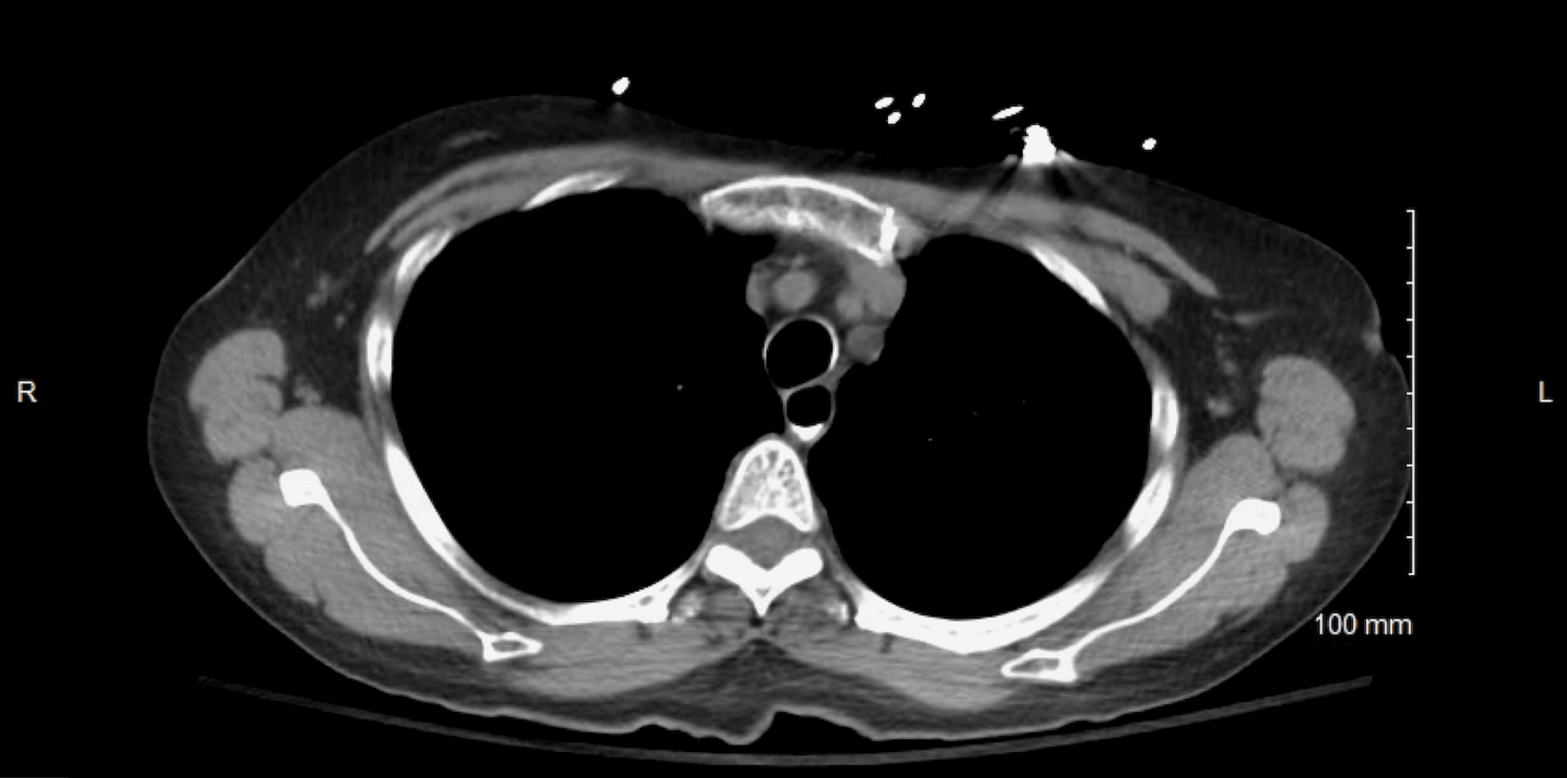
Repeat CT of the chest demonstrating resolution of PM PM, pneumomediastinum
